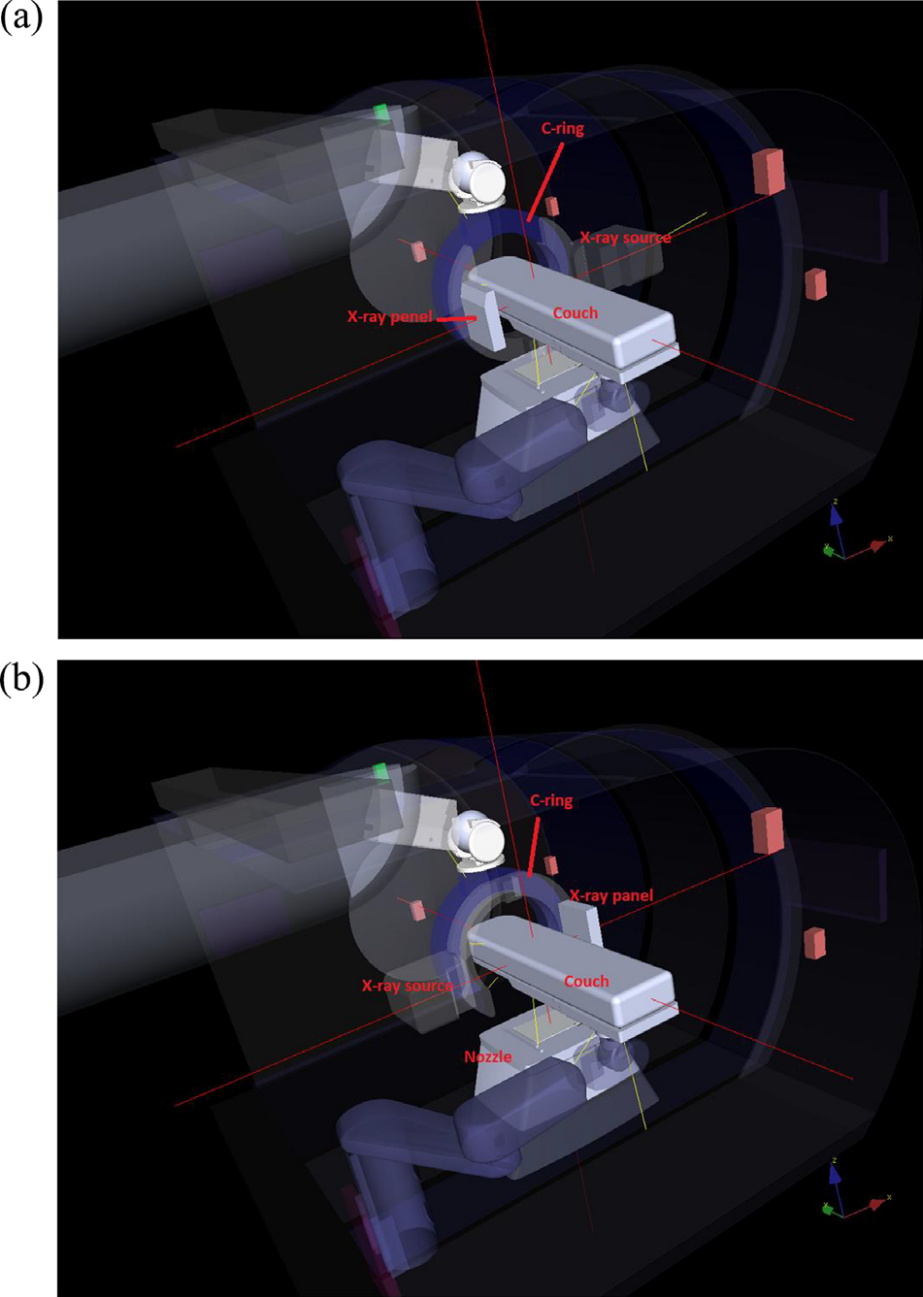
CAS real‐time monitoring model; (a) demonstrates the x‐ray panel on the right side for a 0‐degree couch while the source is on the left side (corresponding to the IEC 61217 fixed reference coordinate system (b) demonstrates the x‐ray on the left side of the 0‐degree couch while the source is on the right side. The CBCT ring is shown in blue. The patient collision envelope is shown atop the couch. The software detects the closest approach based on the modeled components slows down at a 25 cm distance, and stops the motions at 1 cm.
